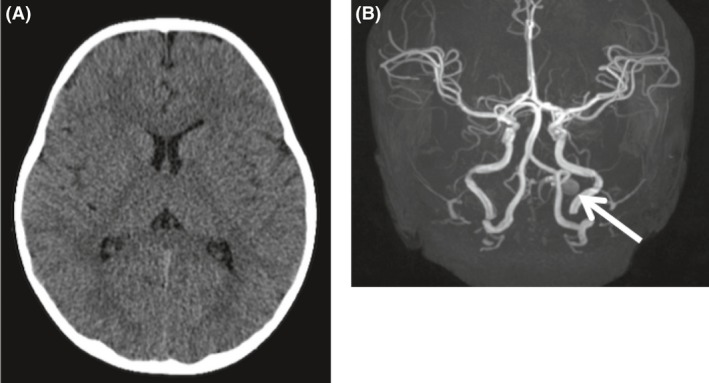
Brain CT (A) and MRI (B) images on the first day. No hemorrhage is detected on the brain CT. (B) The arrow on the brain MRI shows a varix on the left PICA, which was not considered as a cause of the symptoms. CT, computed tomography; MRI, magnetic resonance imaging; PICA, posterior inferior cerebellar artery.
